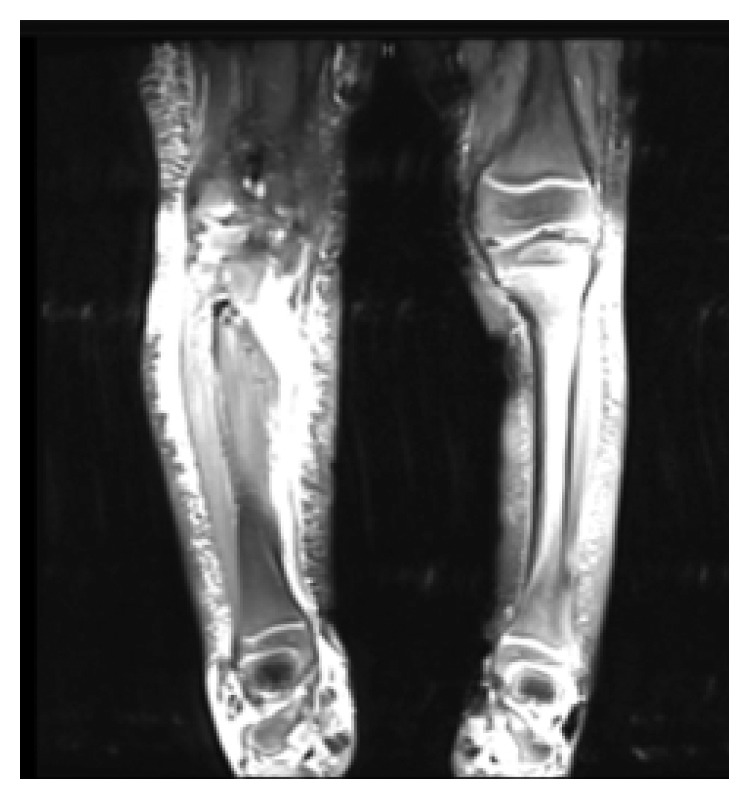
MRI scab showing the deep tissue inflammation and soft tissue edema.
